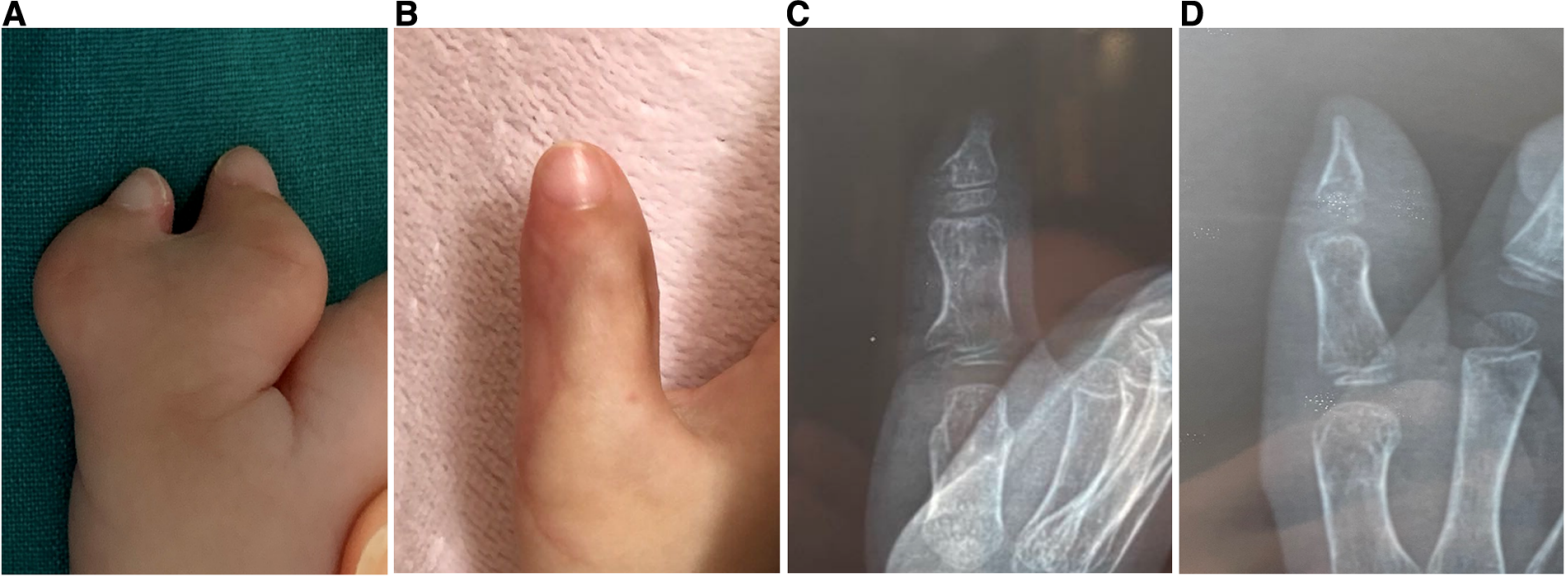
Case 9, (A) preoperative view. (B–D) Postoperative view and radiograph 28 months after none of the nails were combined.
